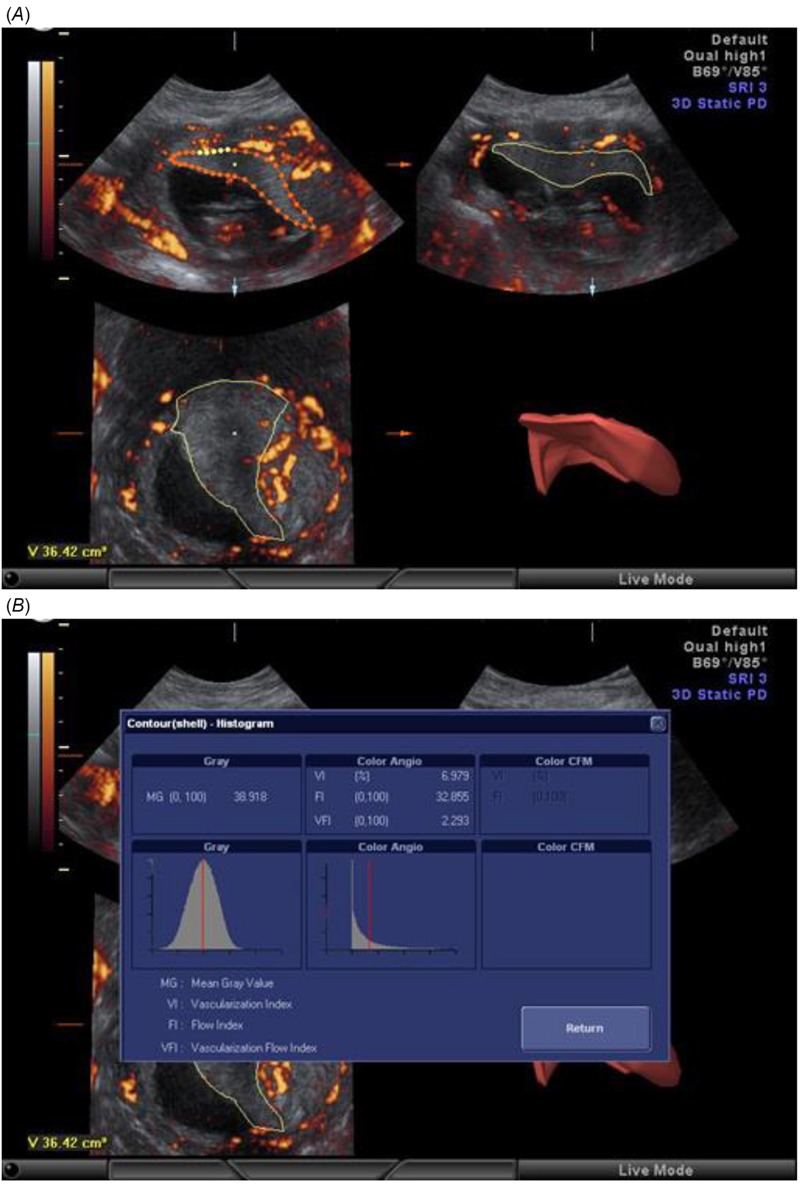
Three-dimensional power Doppler (3DPD) ultrasound. (*A*) Placental capture by 3DPD ultrasonography with the virtual organ computer-aided analysis (VOCAL) method. (*B*) Assessment of placental volume using the rotational technique (VOCAL) and a 3DPD histogram showing the vascular indexes.
